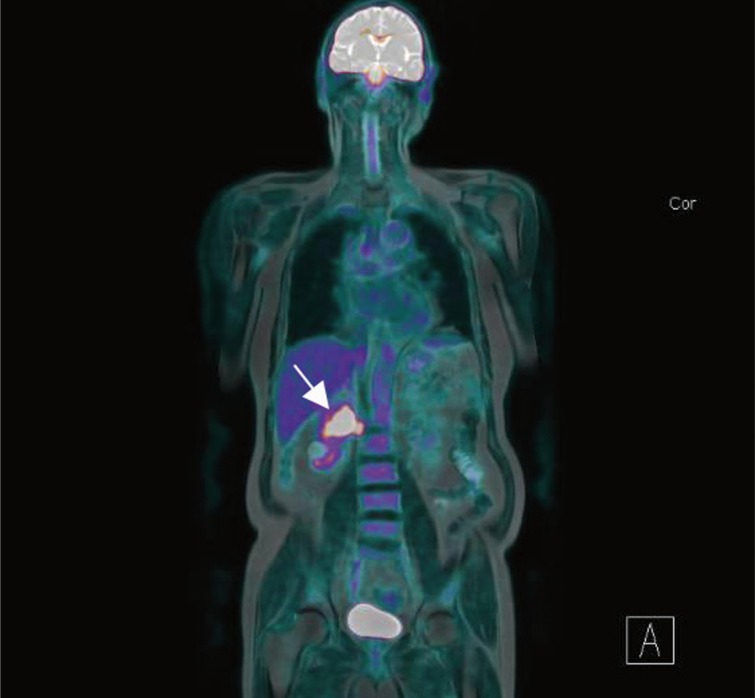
Coronal PET-MRI scan demonstrating a solitary enhancing lesion in the right renal hilum (arrow).
